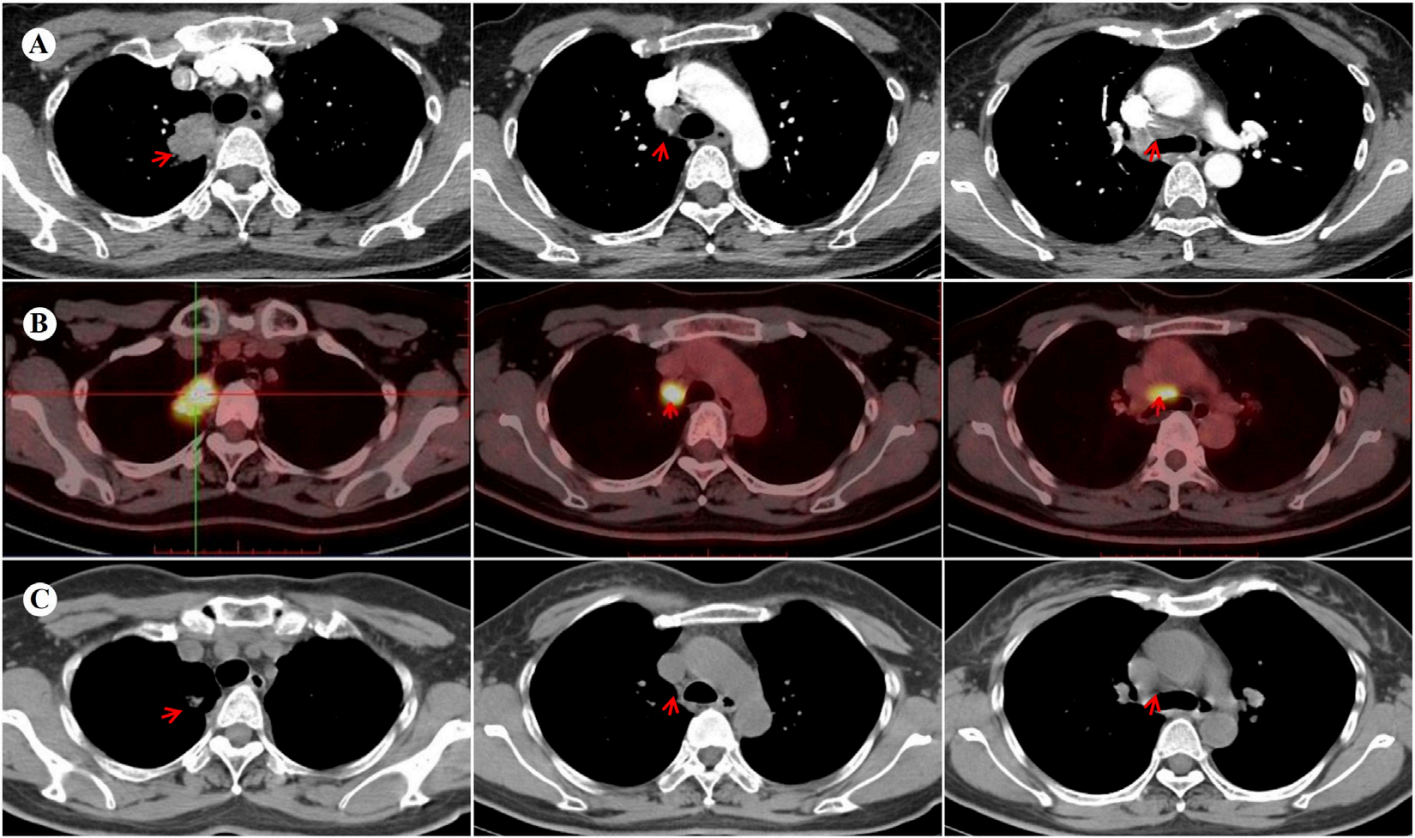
Images before and after neoadjuvant targeted therapy with ensartinib. (A) Contrast-enhanced chest CT at baseline demonstrates a 32 × 24 mm mass with heterogeneous enhancement in the right upper lobe, accompanied by enlarged mediastinal lymph nodes (stations 2R/4R). (B) The baseline PET-CT performed on 21 November 2023 reveals hypermetabolism in the primary lesion (maximum standardized uptake volume [SUVmax] 14.8) and mediastinal lymph nodes (SUVmax 15.5), without distant metastasis. (C) Follow-up lung CT at 3 months post-neoadjuvant therapy shows a significant reduction of the primary lesion (11 × 7 mm) and mediastinal lymph nodes, indicating a partial response (PR) to ensartinib. CT, computed tomography; PET, positron emission tomography.
